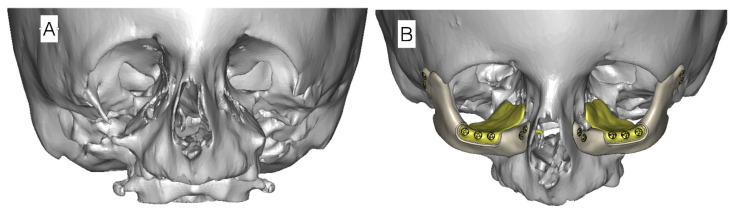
Example of virtual planning of zygomatic bone reconstruction with alloplastic implants. (A) Computed tomography image prior to transplantation in a patient with missing zygomatic bone. (B) CT scan image with planned alloplastic zygomatic bone reconstruction and individual orbital floor recontouring plates.
